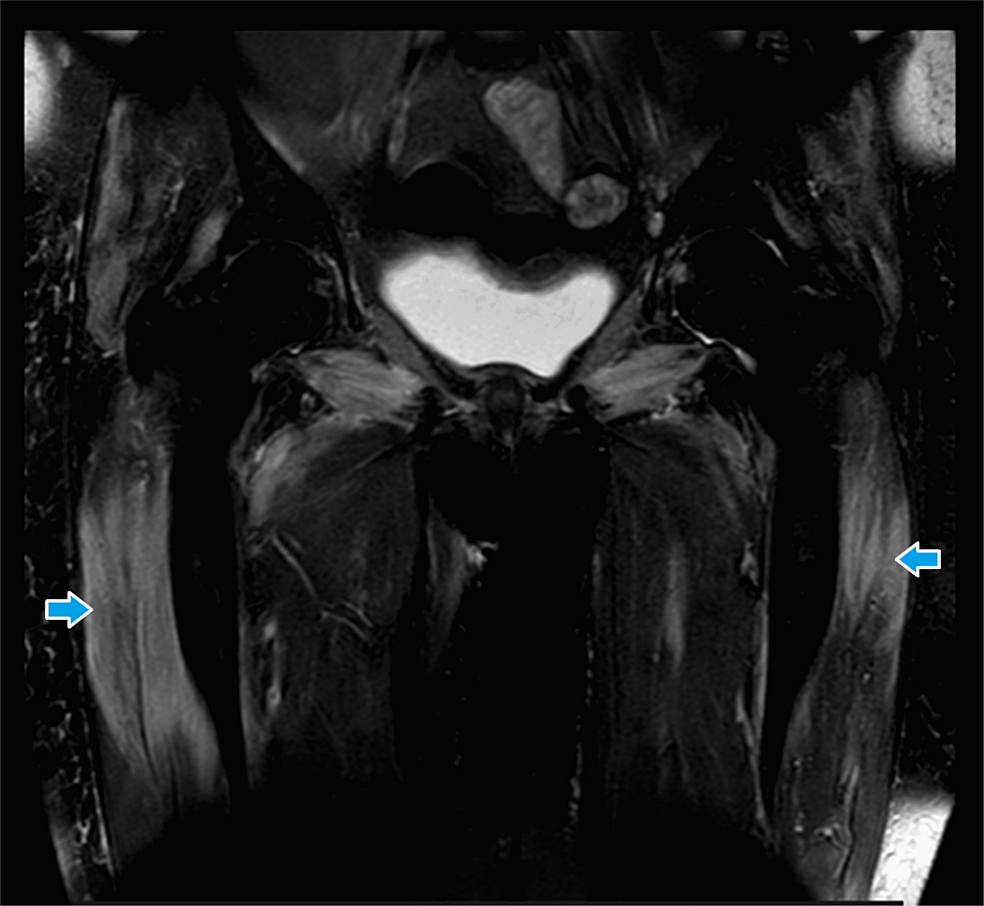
Magnetic resonance imaging (T2-weighted, coronal view) of the thighs showing high signals in muscles.
